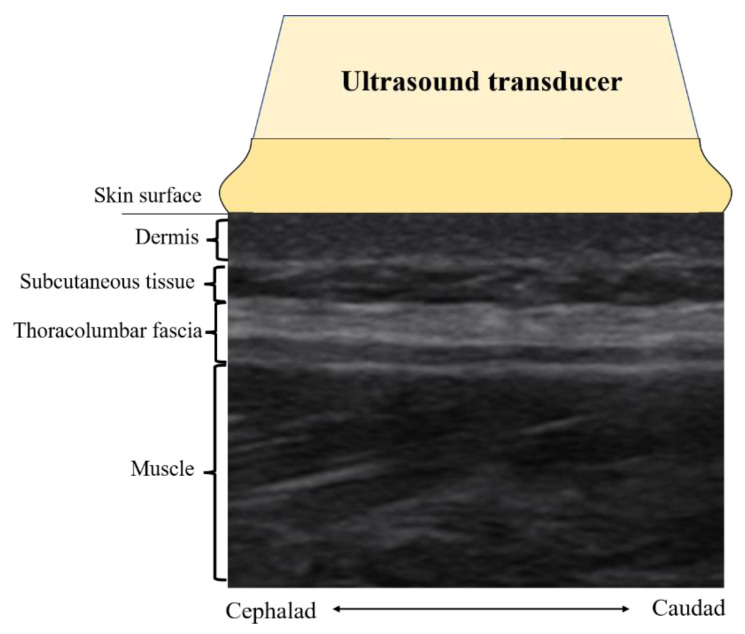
Example of a parasagittal ultrasound image.
